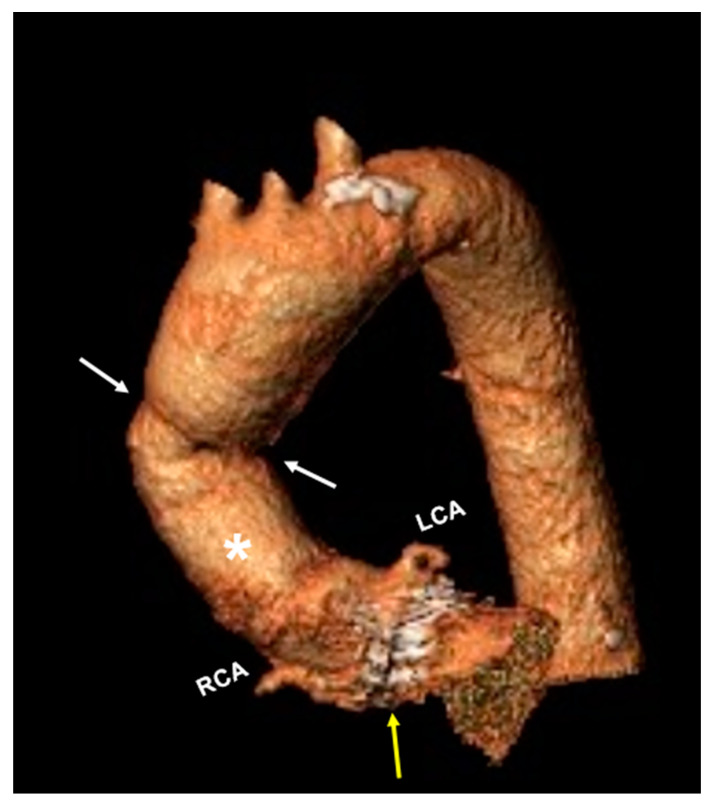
Postoperative CTA examination and three-dimensional reconstruction indicated a successful Bentall procedure with replacement of the ascending aorta (white asterisk), the aortic root, and the aortic valve (yellow arrow) with reimplantation of left and right coronary artery (LCA and RCA). Distal anastomosis is indicated by the white arrows.
